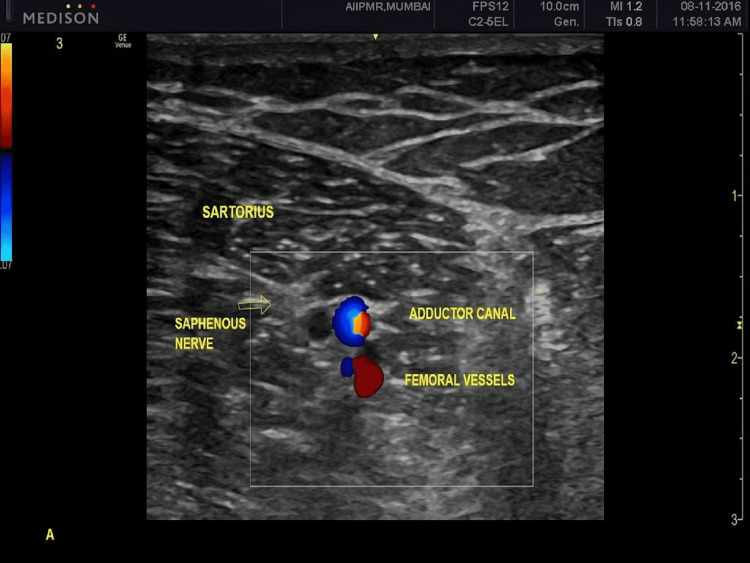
Ultrasound Image Showing the Location of the Saphenous Nerve at Midthigh Level
